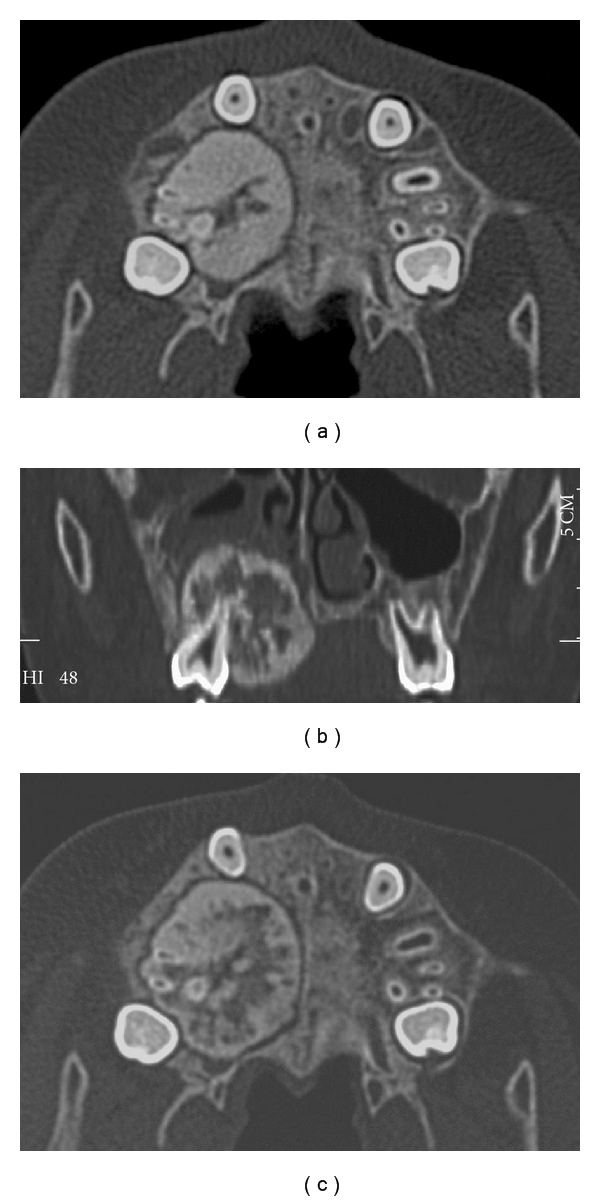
(a) The tumour contained low-density and cemental regions. (b) CT showing penetration into the nasal cavity and maxillary sinus. (c) The tumour showed rapid expansion over 2 months.
